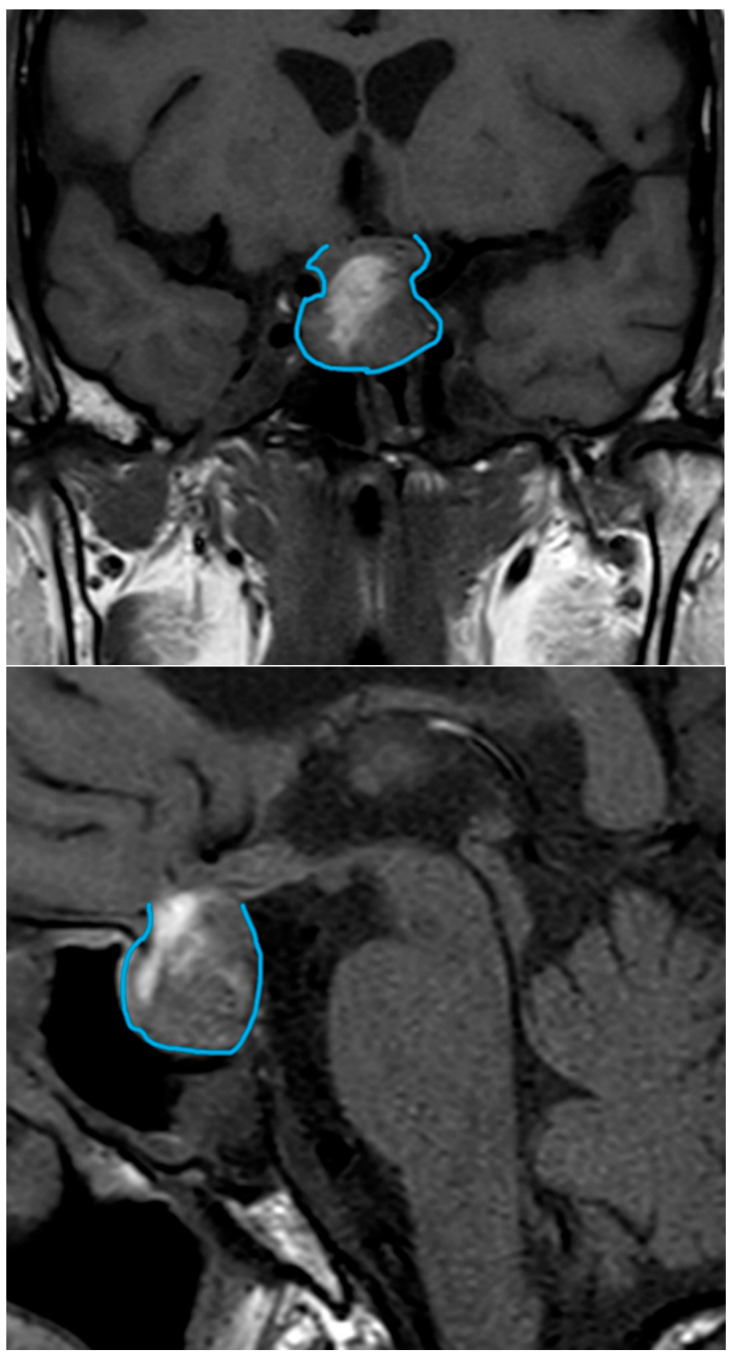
MRI, July 2018. Macroadenoma (20 × 20 × 18 mm) with signs of pituitary apoplexy and an invasion of not only the optic chiasm but also the hypothalamus and partially the left cavernous sinus. The tumor is delineated in all imaging studies. MRI, magnetic resonance imaging.
